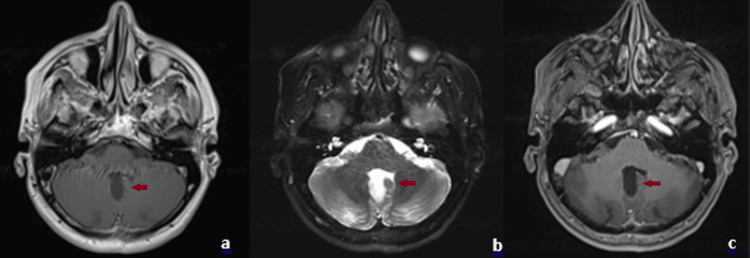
Postoperative axial magnetic resonance imaging (MRI) scans of the posterior fossa (a) T1-weighted image showing postoperative changes without residual tumor. (b) T2-weighted image demonstrating regression of perifocal edema. (c) Contrast-enhanced T1-weighted image revealing no pathological enhancement, confirming gross total resection.
